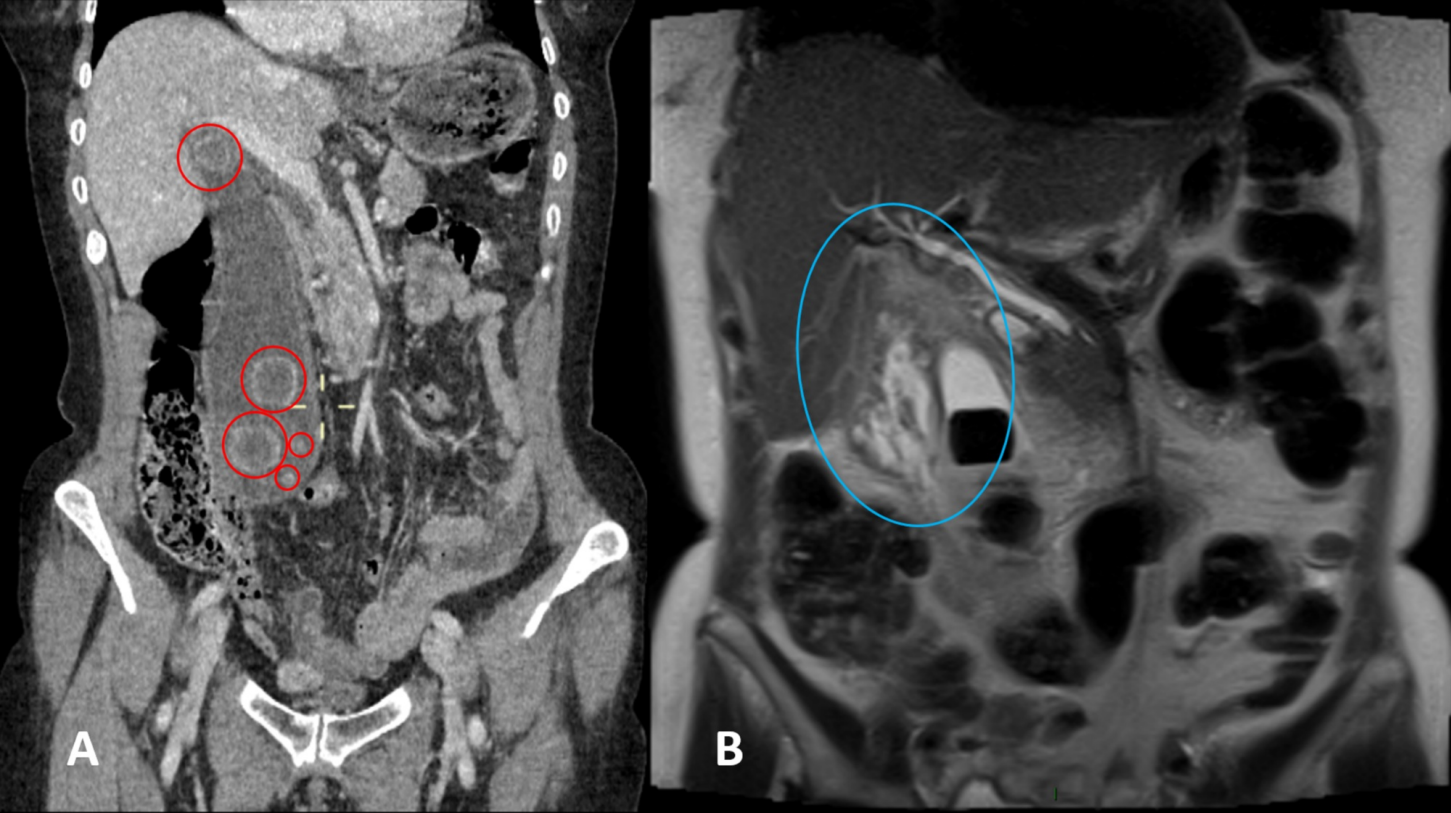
Abdominal computed tomography (CT) scan Abdominal computed tomography showing a markedly enlarged gallbladder containing several stones measuring (red circles) 19.5 x 5.4 x 5.6 cm in the (A) craniocaudal, transverse and anteroposterior dimensions and in the (B) anatomical pelvis. Significant edematous changes in the area between the distended gallbladder with stones and the liver suggested gallbladder perforation (blue circle).
